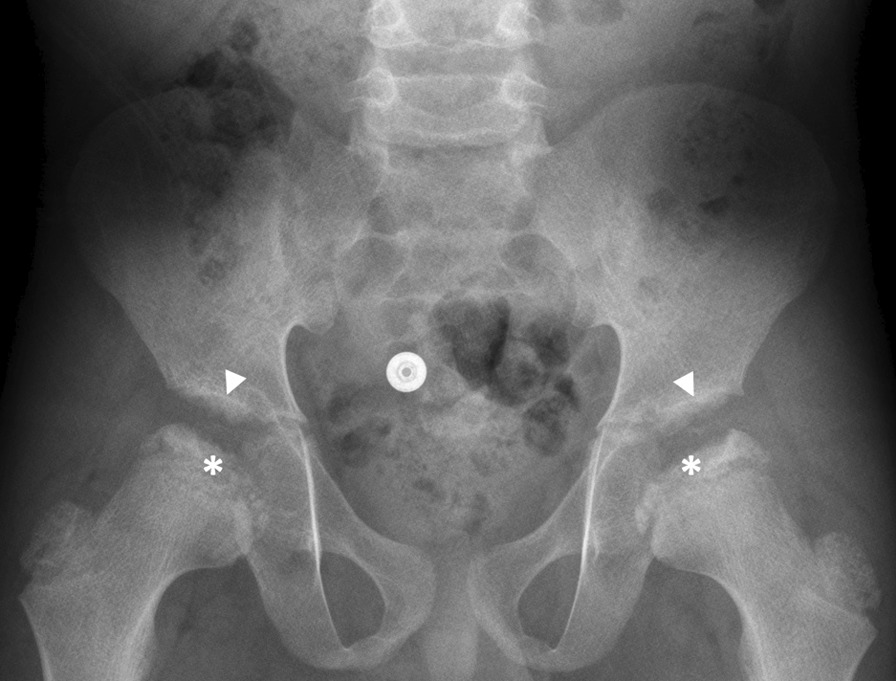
Anteroposterior radiograph of hips and pelvis in Patient 1 at 6 years of age shows bilateral sclerotic, fragmented, and flattened proximal femoral epiphyses (asterisks), irregular acetabula (arrowheads), and coxa valga deformities. The patient was initially diagnosed with Legg–Calve–Perthes disease; in retrospect, acetabular irregularities and coxa valga would not be typical in this condition
